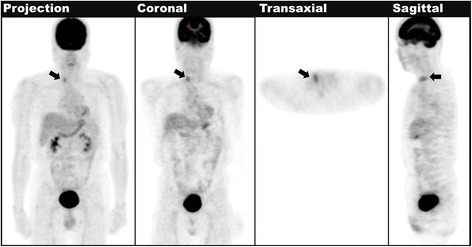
The first time FDG-PET scan of the patient of resected colorectal cancer with elevated CEA level (68 µg/L) showed focal FDG uptake in the right thyroid lobe (*arrow*)
